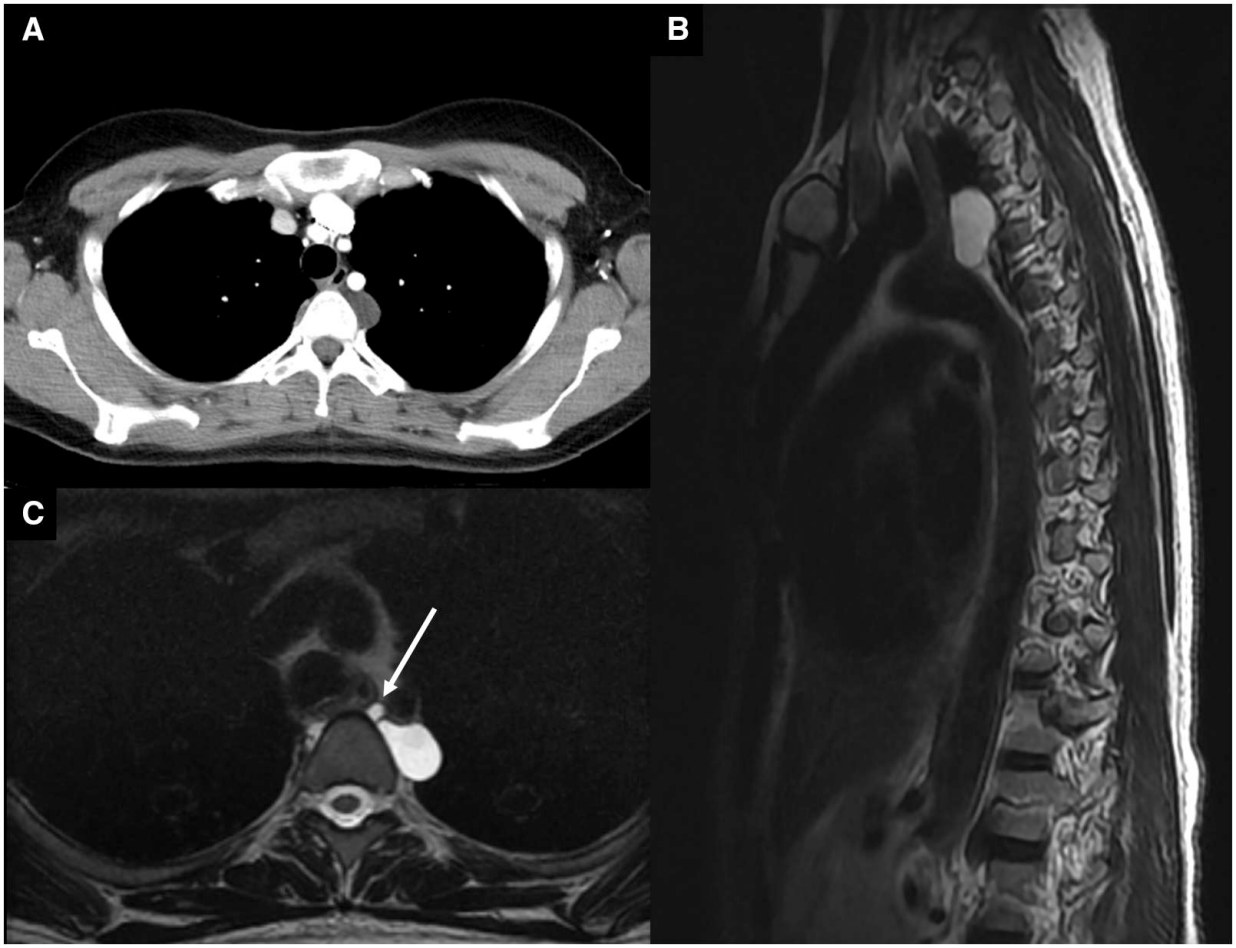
(a) Computed tomography of mediastinal Mullerian cyst. (B) T2-weighted MRI sagittal view showing a cyst between the left subclavian artery and the descending aorta. (C) T2-weighted MRI axial view demonstrating cyst and thoracic duct (arrow)
